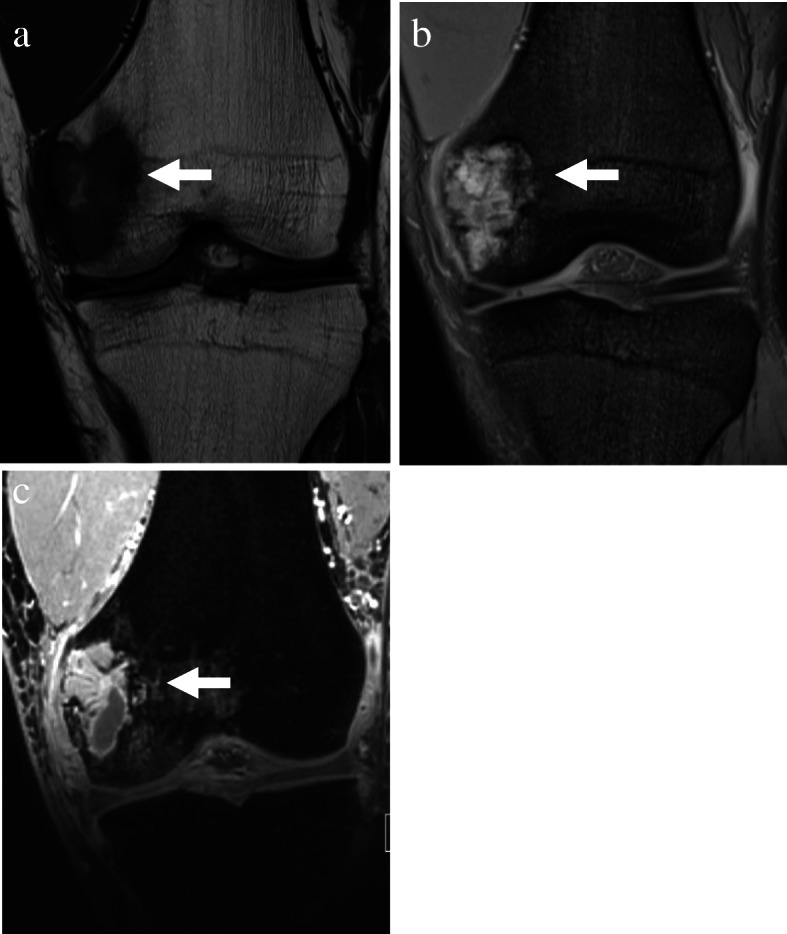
Magnetic resonance imaging shows an ill-defined mass lesion in the left distal femur. Ill-defined mass lesion appears (a) hypointense on T1-weighted image and (b) hyperintense and isointense on T2-weighted image. c T1-weighted image with gadolinium shows primary enhancement at mass lesion excluding small non-enhancement area
